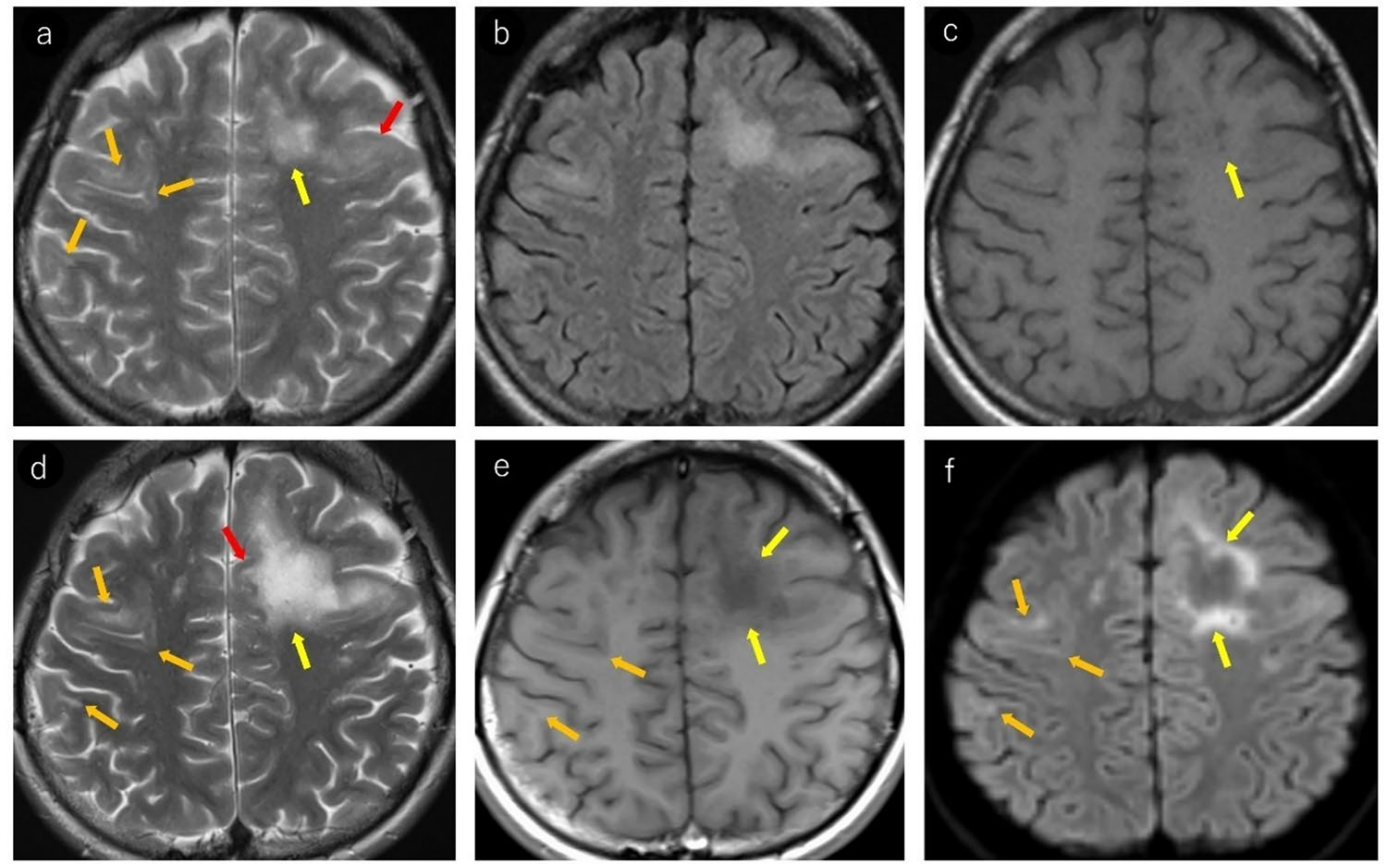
Woman in her 30 s with a known medical history of HIV infection presented with symptoms of mild paralysis in her right lower extremity and exhibited mild articulation disorder. Notably positive JCV–PCR in the cerebrospinal fluid, confirmed a diagnosis of PML. Initial MRI findings (a–c): a T2-weighted imaging reveals heterogeneous hyperintense lesions in the left frontal lobe extending from the corticomedullary junction to the subcortical white matter (yellow arrows). Subtle hyperintensity is also noted in the left frontal cortex (red arrows). Subtle linear T2 hyperintense lesions are observed at the corticomedullary junction of the right frontal and parietal lobes (orange arrows). b FLAIR image demonstrates more conspicuous bilateral frontal and right parietal lesions, enhancing their detectability. c Left frontal lesion appears as a mildly heterogeneous hypointense area on T1-weighted images (yellow arrows). Right frontal and parietal lesions were not identifiable. Follow-up MRI after a month (d–f): d linear T2 hyperintense lesions at the corticomedullary junction of the right frontal and parietal lobes show slight progression in size and conspicuity (orange arrows). The left frontal lesion expanded, exhibiting sharp margins on the cortical side (red arrows) and ill-defined borders on the white matter side (yellow arrows). e On T1-weighted imaging, the left frontal lesion demonstrates heterogeneous hypointensity with a central CSF-like signal intensity (yellow arrows). Corticomedullary junction lesions in the right frontal and parietal lobes also show subtle hypointensity (orange arrows). f Left frontal lesion exhibits a rim and core pattern on diffusion-weighted imaging, with a hyperintense periphery and a hypointense center (yellow arrows). Corticomedullary junction lesions in the right frontal and parietal lobes showed subtle linear hyperintensity (orange arrows) and were readily identifiable
